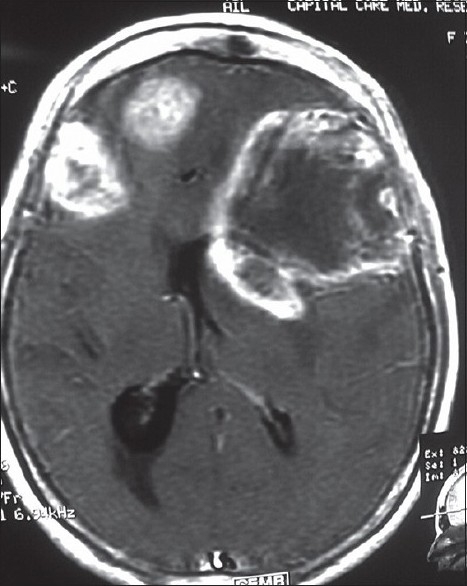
T1WI axial MRI brain of an orchard worker showing multicentric glioma
